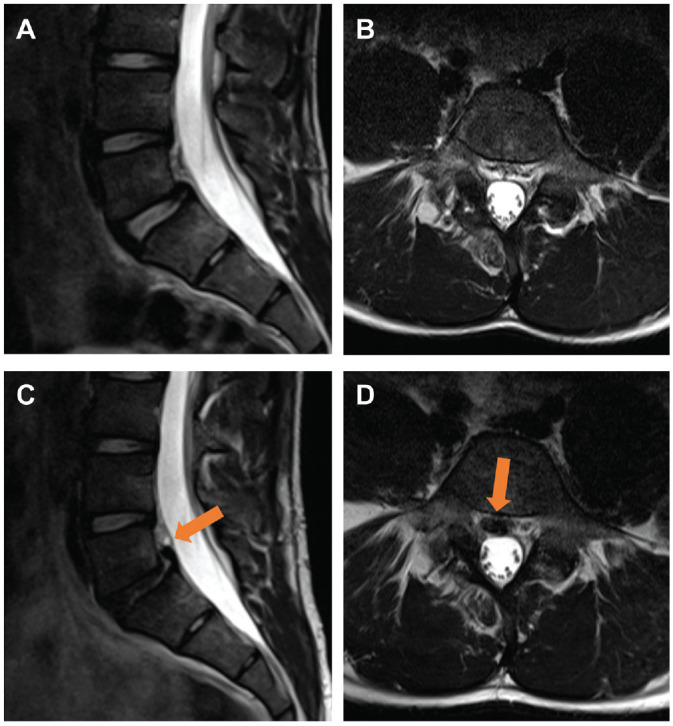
Sagittal Dixon and transverse T2-weighted 3-T magnetic resonance imaging scans of the lumbar spine of an 18-year-old competitive alpine skier (A and B, respectively) at baseline and (C and D, respectively) at the 48-month follow-up. Note that the progression of disk degeneration led to new herniation and the formation of a sequester (arrows), most likely due to the continuous high load applied during alpine skiing. Additionally, anterolisthesis at the L5-S1 level is evident, which is a consequence of the patient's spondylolysis.
